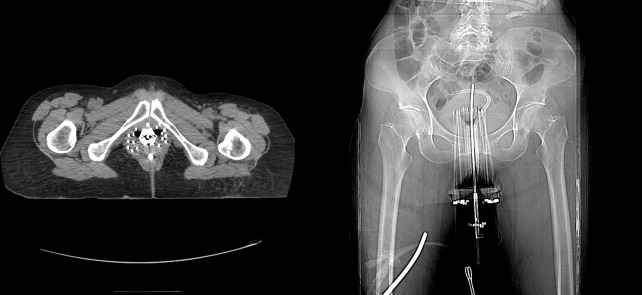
Planning CT for interstitial high-dose-rate brachytherapy following neoadjuvant chemoradiotherapy. Axial and scout images taken immediately following the placement of interstitial needles through the cervix with a central intra-cavitary tandem for high-dose-rate brachytherapy.
